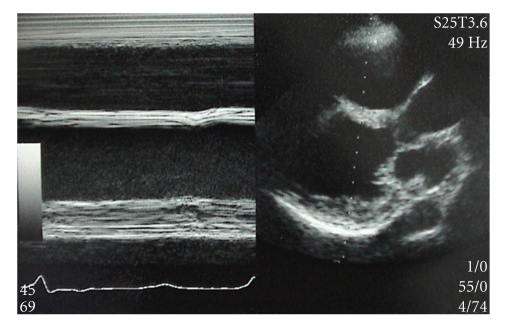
Echocardiography shows biventricular enlargement and diffuse hypokinesis of the left ventricle without wall thinning.
